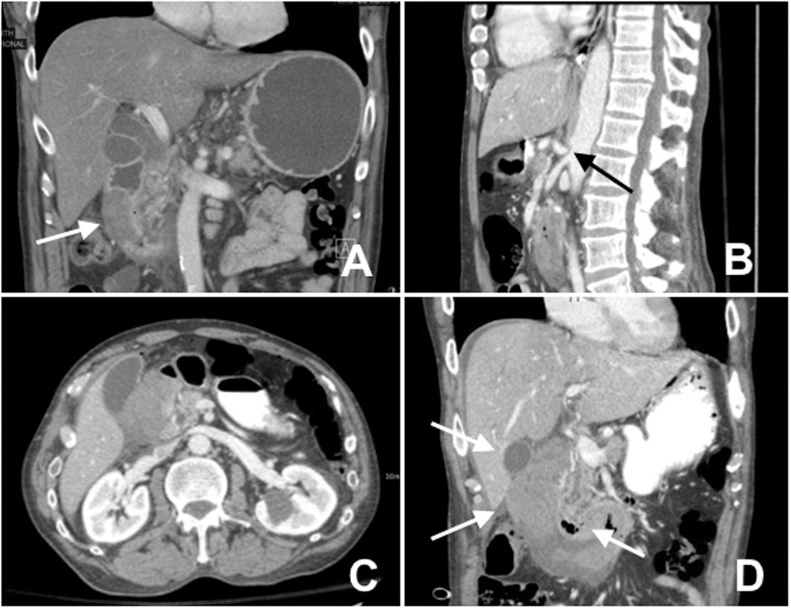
(A) Initial CT scan of patient MM demonstrates a hematoma in the lateral wall of the duodenum. (B) Severe celiac artery stenosis likely from median arcuate ligament. (C) Enlarged pancreaticoduodenal arteries that may have contributed to the formation of the patient’s hematoma. (D) CT performed after patient clinically deteriorated demonstrated a duodenal hematoma extended from D1–D3 with extraluminal gas suggested a perforation.
